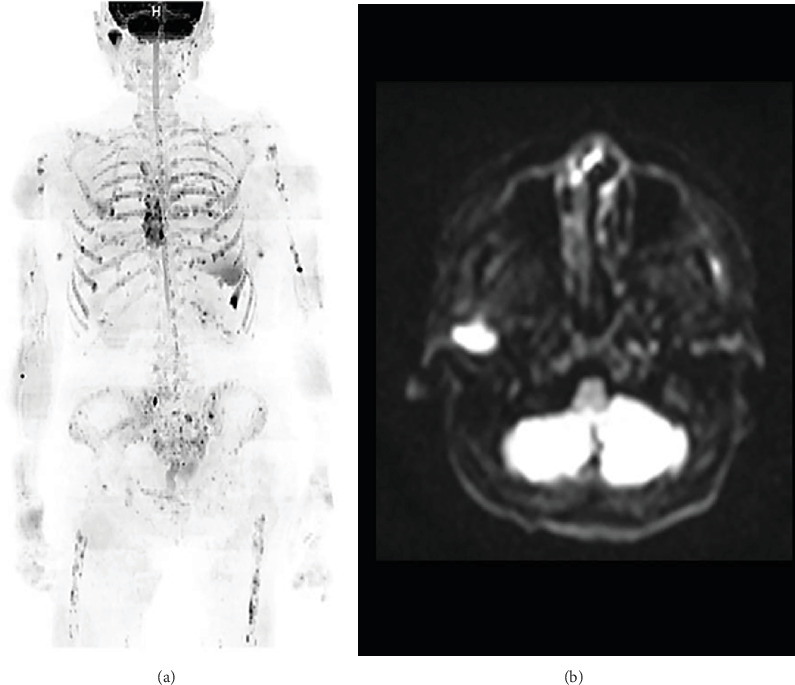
Magnetic resonance imaging (MRI). (a) On whole-body diffusion-weighted imaging with background body signal suppression, diffusion-restricted areas are observed systemically, including in the right mandibular condyle, sternum, ribs, humeri, femurs, and iliac bones. (b) Signal hyperintensity is observed in the right mandibular condyle on the apparent diffusion coefficient map of diffusion-weighted imaging.
